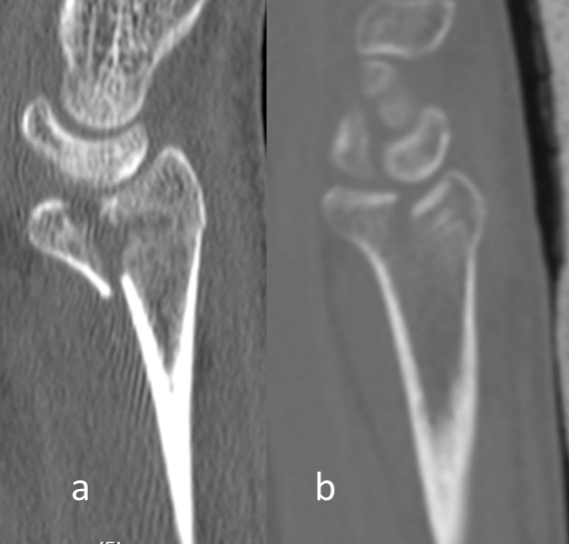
a: CT in sagittal plane of a distal radius fracture with a palmar extending frontal split. b: CT in sagittal plane of a distal radius fracture with a dorsal extending frontal split. Difference between frontal splits.
